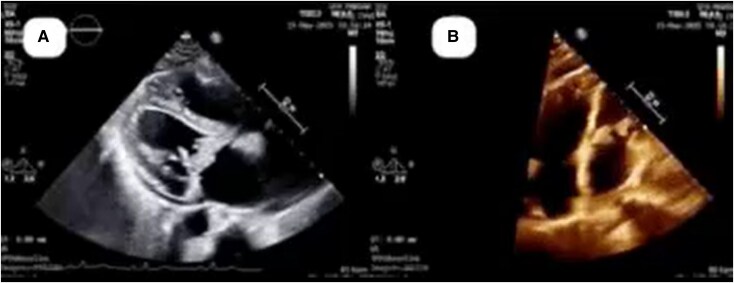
Transthoracic echocardiography; (GIF *A*): parasternal long axis view, showing large masses causing mitral valve obstruction. (GIF *B*): Apical 4 chambers view showing right ventricular dilatation (42 mm at the base) and dysfunction, and biatrial enlargement.
